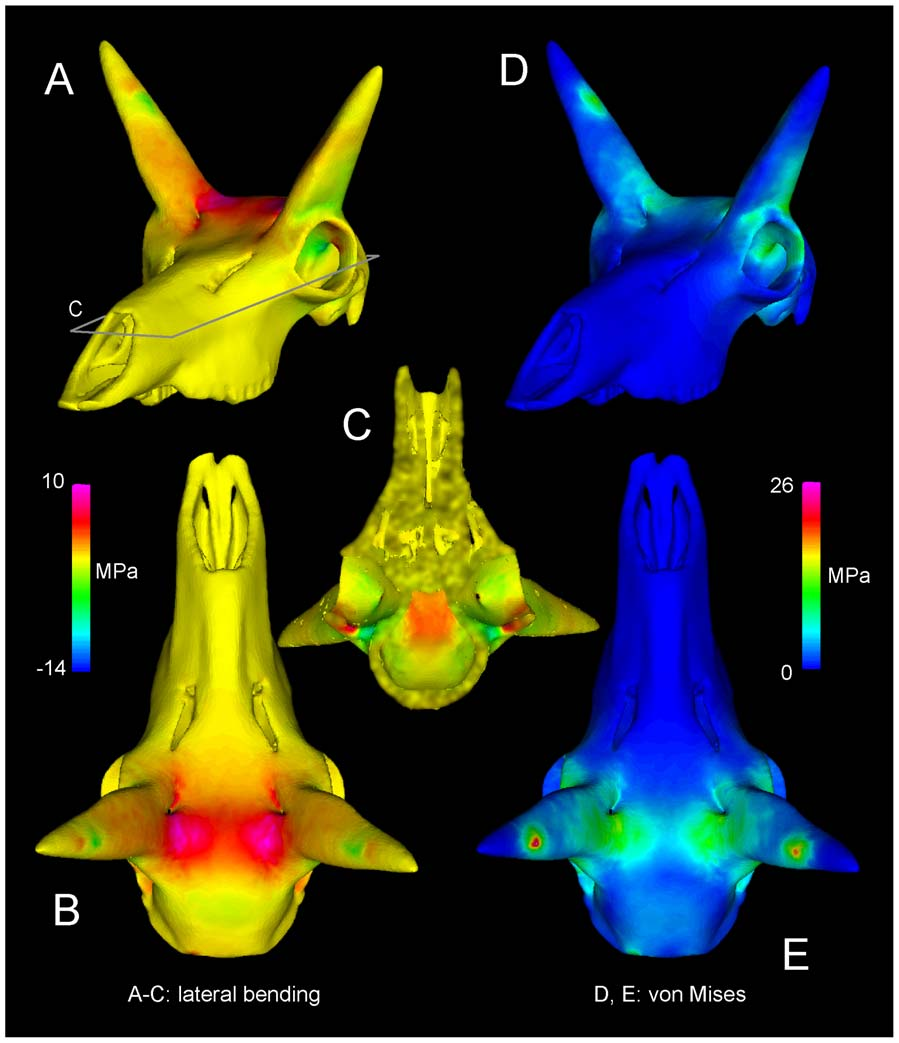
Head-strike stresses in the pronghorn (UCMZ M 1989.61). Stresses in *Antilocapra americana*, primarily from mediolateral bending (A–C) and consolidated as von Mises stress (D, E). C is a ventral view of the cranium sectioned in the plane shown in A. Relatively high tensile stresses occur at the base of the pronghorn cores (A, B) and roofing the endocranial cavity (C), where CT reveals dense compact bone (Figure 8).
